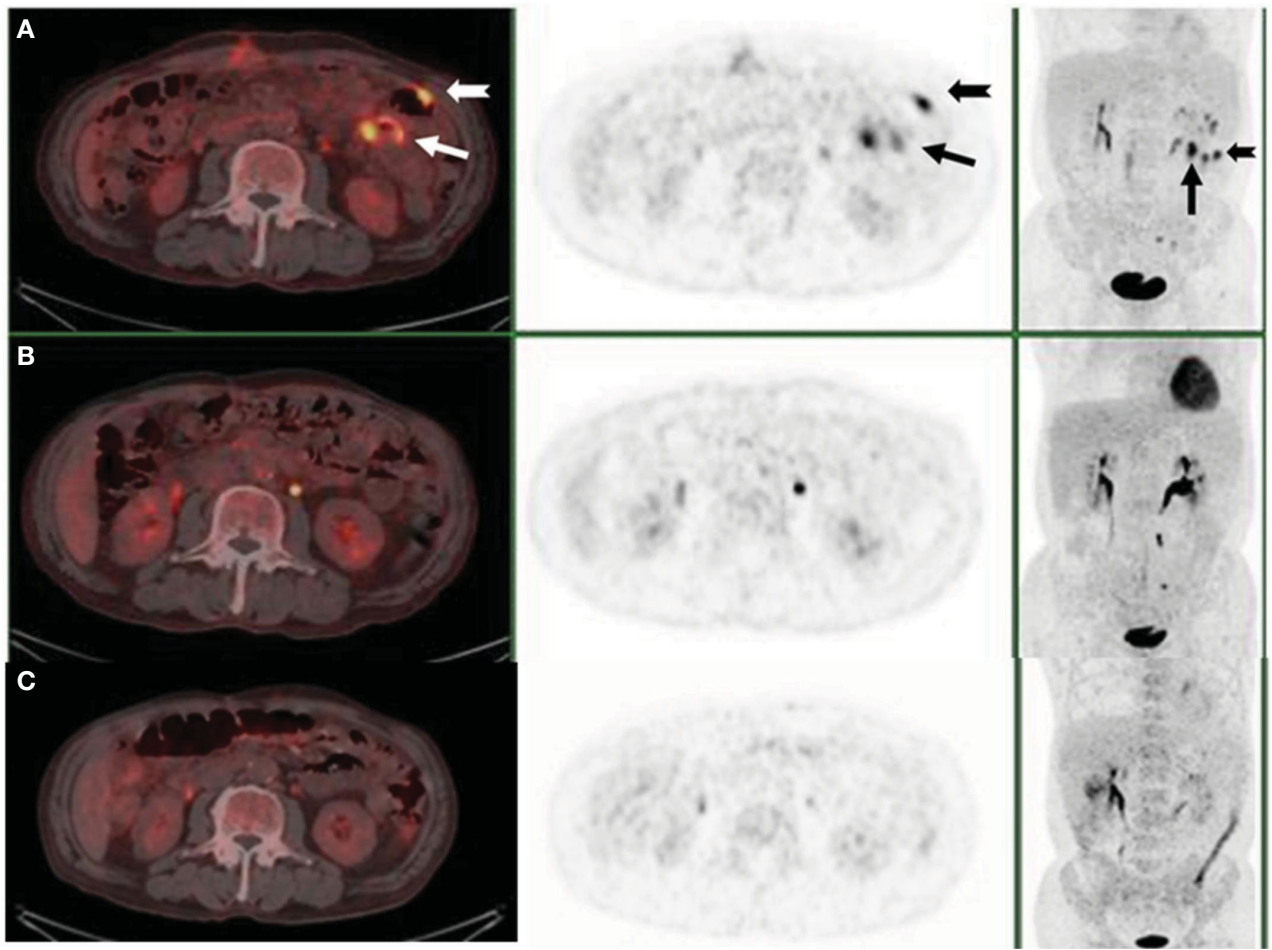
PET/CT scan after small bowel resection (A), three cycles of R-CHOP (B), and five months after treatment (C). The arrows demonstrated the lesions where abdominal masses disappeared.
